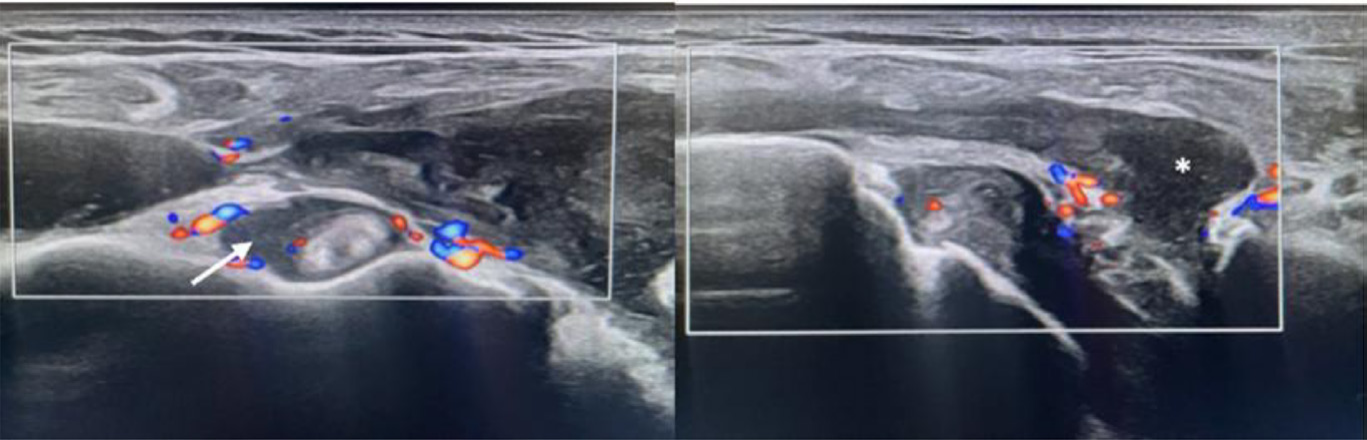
Ultrasound scan of the left shoulder showing subacromial-deltoid bursitis (asterisk) and tenosynovitis of the long biceps tendon (arrow).
